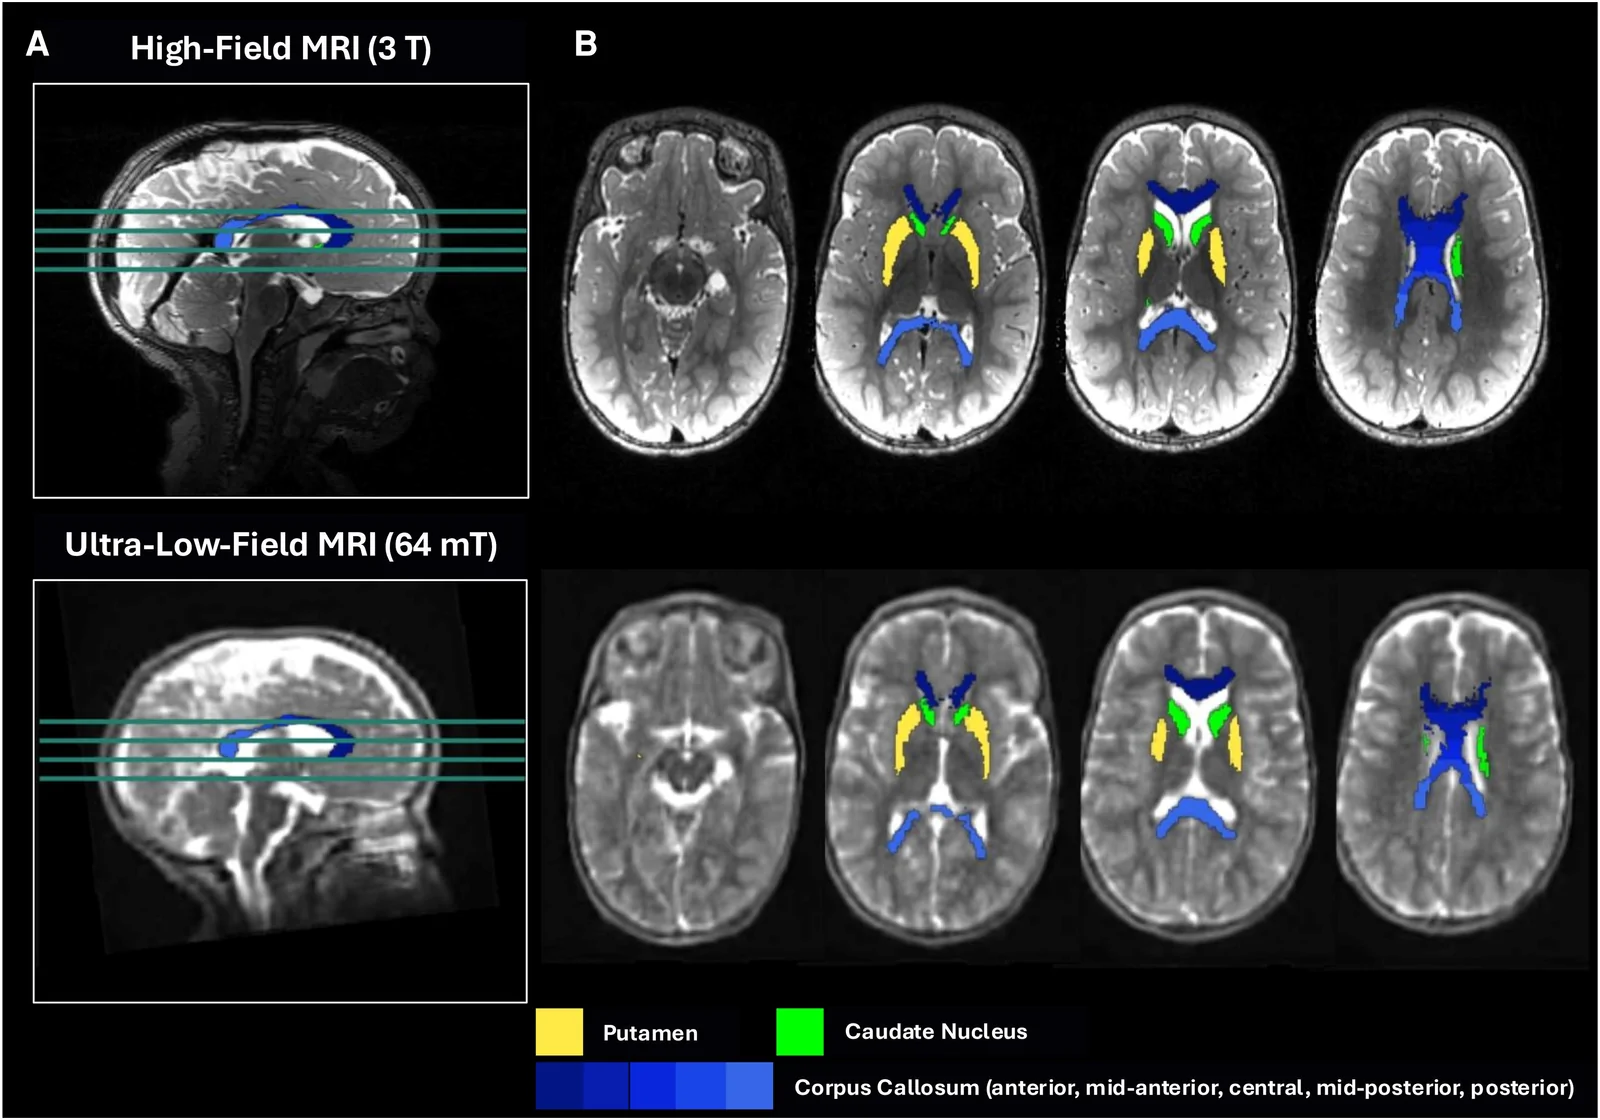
Segmentation overlay of brain regions associated with antenatal maternal anaemia in an infant at 12 months of age scanned using HF (3 T) and ULF (64 mT) MRI. The brain images were created using MRIcroGL software (Version 15.0.1). (A) Sagittal view of the brain demonstrating slices from inferior (bottom) to superior (top). (B) Corresponding axial slices with segmentation overlay for brain regions of interest from inferior (left) to superior (right).
